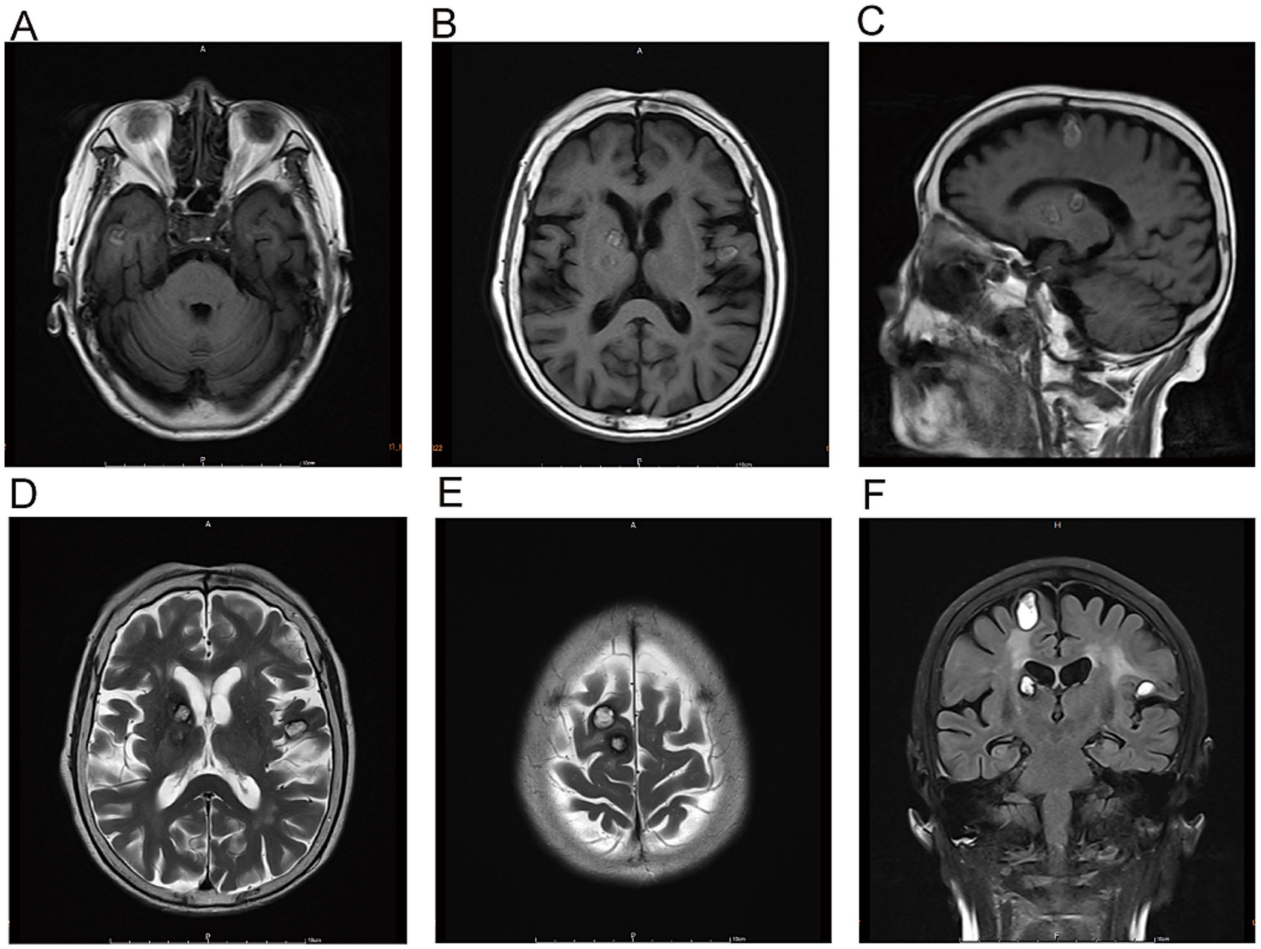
Multiple lesions are noted in the right frontal-temporal lobe, basal ganglia, and left frontal lobe, with perilesional edema. Some lesions have decreased in size compared to previous findings, and the edema has partially resolved. On March 30, the MRI scans demonstrated multiple lesions and perilesional edema in the right frontotemporal lobe, basal ganglia, and left frontal lobe. Specifically, the lesions and edema were partially reduced compared with previous findings on T1-weighted images (Panels A–C), T2-weighted images (Panels C, D), and T2-FLAIR sequence (Panel F).
